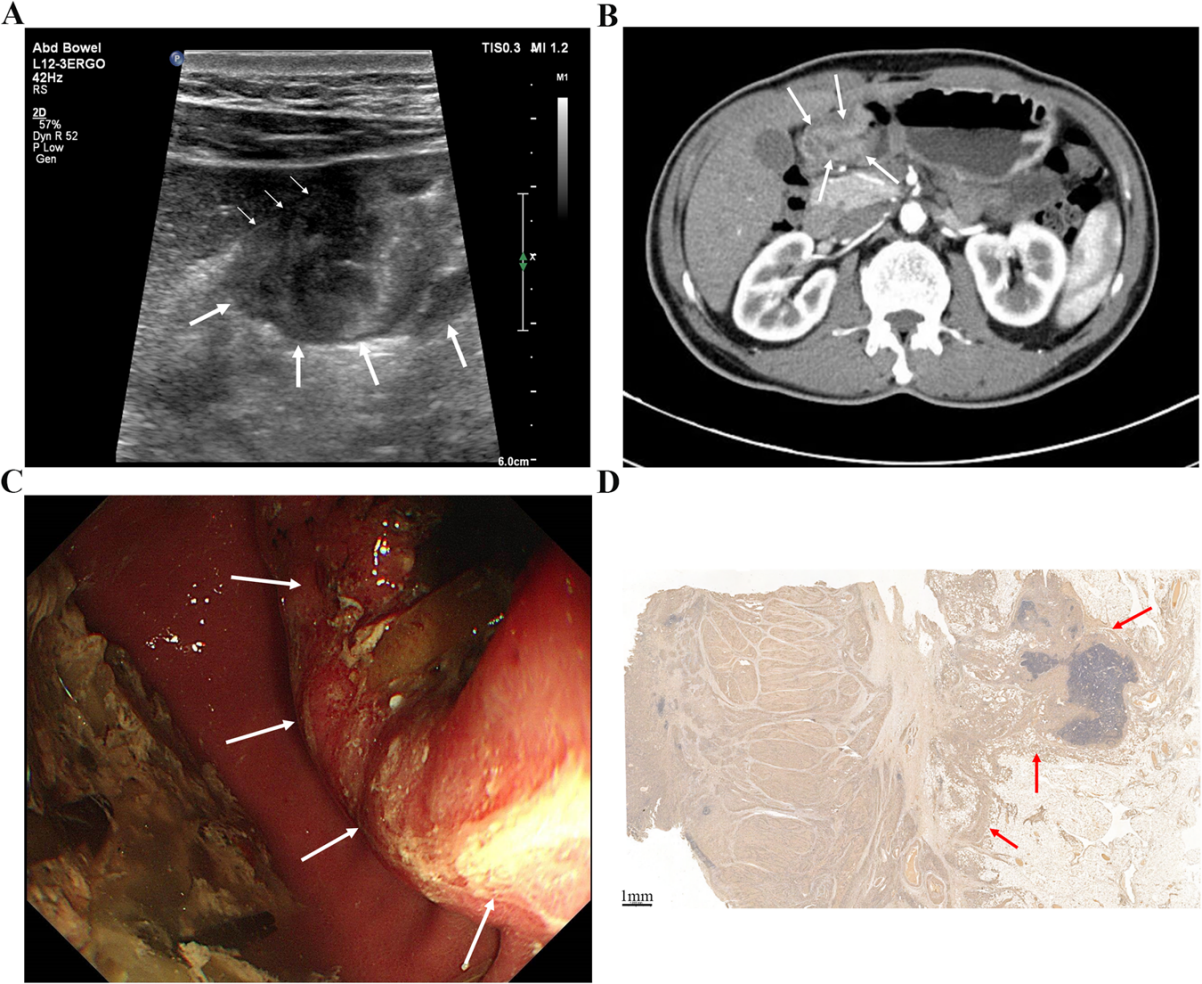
A An approx 55-year-old man underwent gastric oral contrast ultrasonography, revealing full-thickness thickening of the gastric wall (cT4) at the gastric antrum (white thick arrows), with irregular shape, low internal echo, destruction of the gastric wall, irregular serosa echo line, gastric peristalsis stiffness, and unclear boundary with liver tissue (white thin arrows). B The tumor showed local thickening and moderate enhancement, indicating invasion of the whole layer (cT4) of the gastric antrum on enhanced computed tomography. C Electronic gastroscopy found a protrusion ulcer lesion on the gastric antrum, with a white moss-covered center. D The pathological result showed that the tumor invaded the whole layer of the gastric wall and the serosa (pT4) (scale bar: 1 mm)
